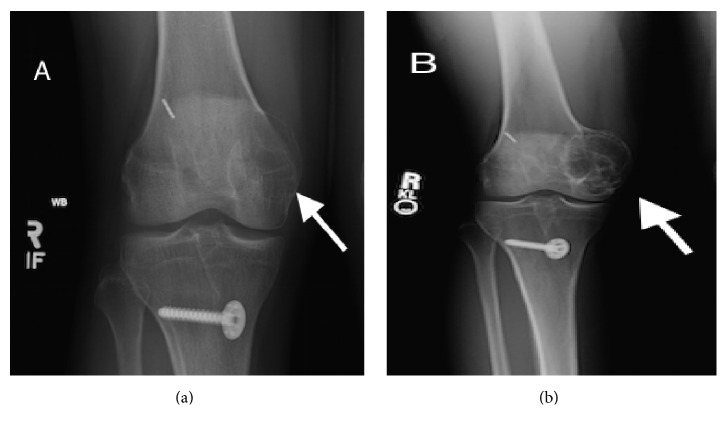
Frontal view radiographs of the right knee obtained (a) 2 years and (b) 4 years after initial imaging demonstrate a closed physis with progressive enlargement of a well-circumscribed, nonsclerotic, eccentric lucent lesion, as indicated by the arrows, at the medial femoral condyle abutting the articular surface.
